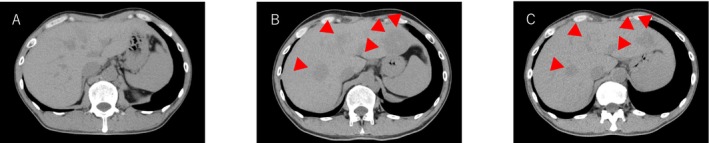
CT images of the present case: (A) Before the start of BEP treatment, (B) At the time of BEP discontinuation, showing evident liver metastasis, (C) 3 months after the initiation of mitotane, demonstrating a significant reduction in liver metastasis.
